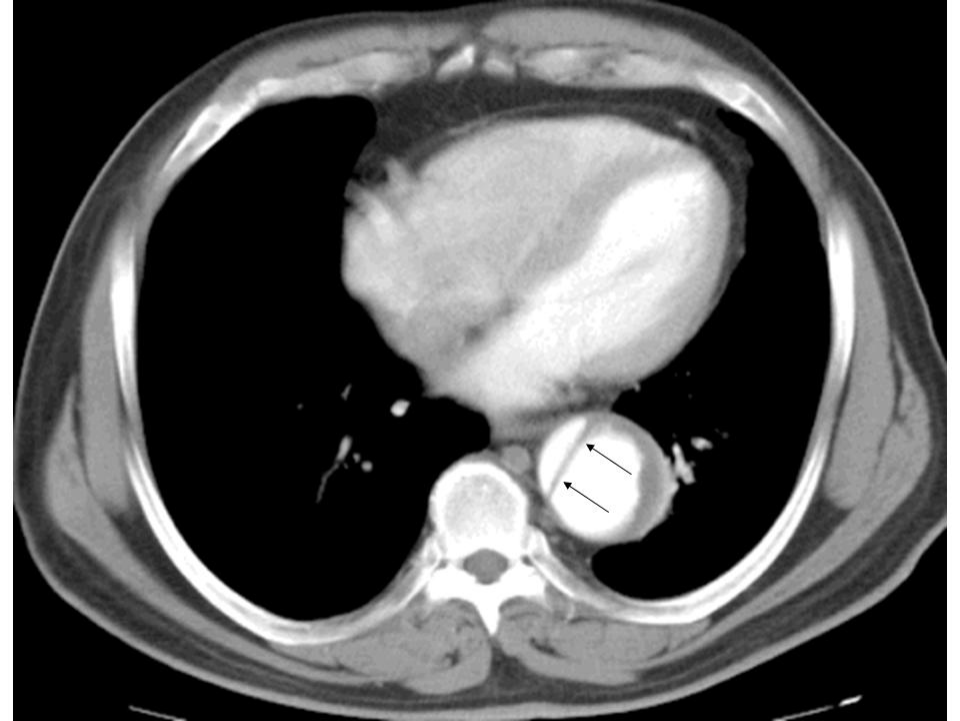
Contrast enhanced CT of the chest obtained in a 67 years old hypertensive male who presented with acute central chest pain shows aortic dissection involving the descending thoracic aorta. Arrows point to the intimal flap. It is important to appreciate that the size and appearance of cardiac chambers on a contrast enhanced image depends on the amount of contrast present in a given chamber at the time of acquisition of the image and is influenced by factors likes volume and speed of injection of the of contrast, time difference between injection of contrast and acquisition of image, cardiac output of a given subject and type of CT scanner
